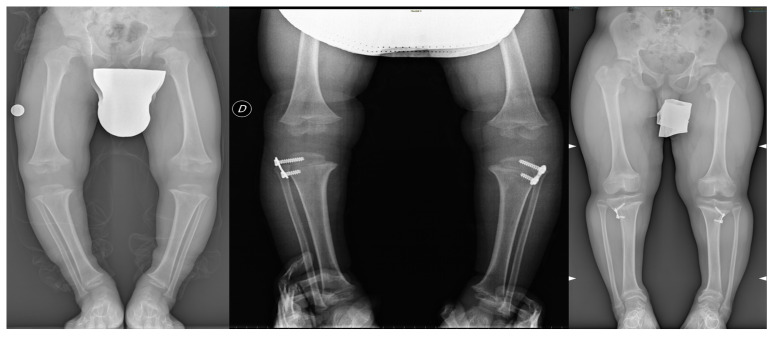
Immediate post-operative and 8-year follow-up radiographs of a 4-year-old male patient with achondroplasia (Ferrara Simone). The images demonstrate good correction of the mechanical axis and successful surgical outcome.
